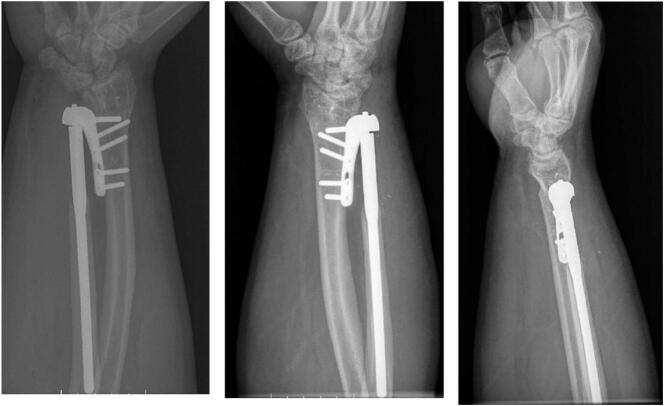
Anteroposterior (AP), lateral, and oblique 6 month post-operative radiographs showing the Scheker prosthesis in place without evidence of loosening or hardware complication. The site of the DRUJ osteotomy is seen without evidence of radio-lunate instability.
